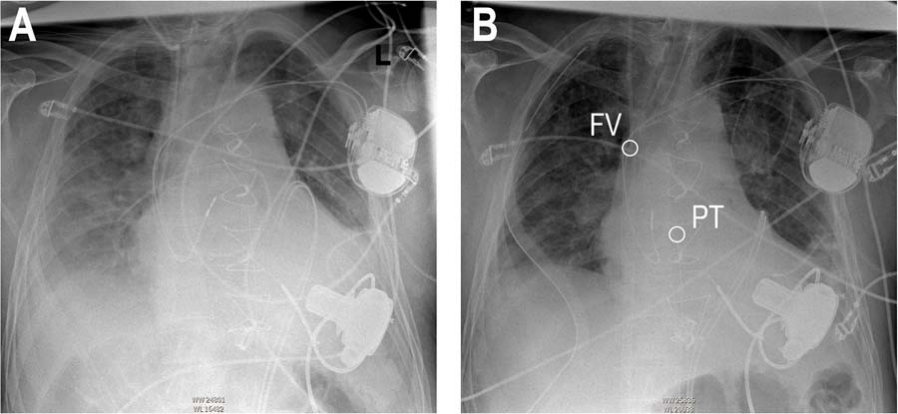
Anteroposterior thoracic radiography after LVAD implantation (A). Anteroposterior thoracic radiography after RVAD cannulation of the PT (B). Tip of cannula in the right FV, tip of cannula in the PT.
